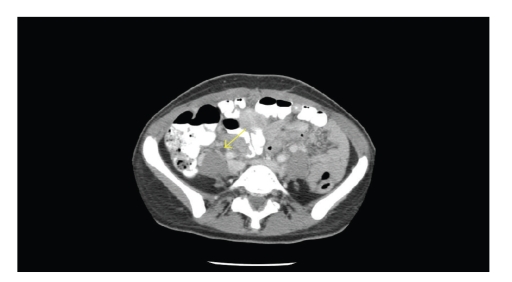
A CT scan of the abdomen showing hyperdense area adjacent to the pancreas and consistent with hemorrhagic pancreatitis.
